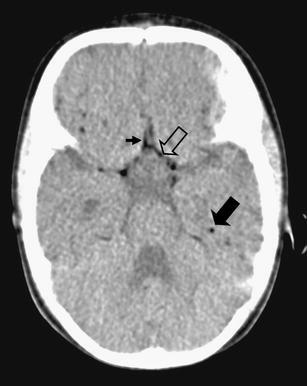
CT at the level of the circle of Willis showing air within the circle of Willis (*open arrow*) anterior cerebral artery (*small arrow*) and peripheral cerebral arteries (*solid arrow*)
